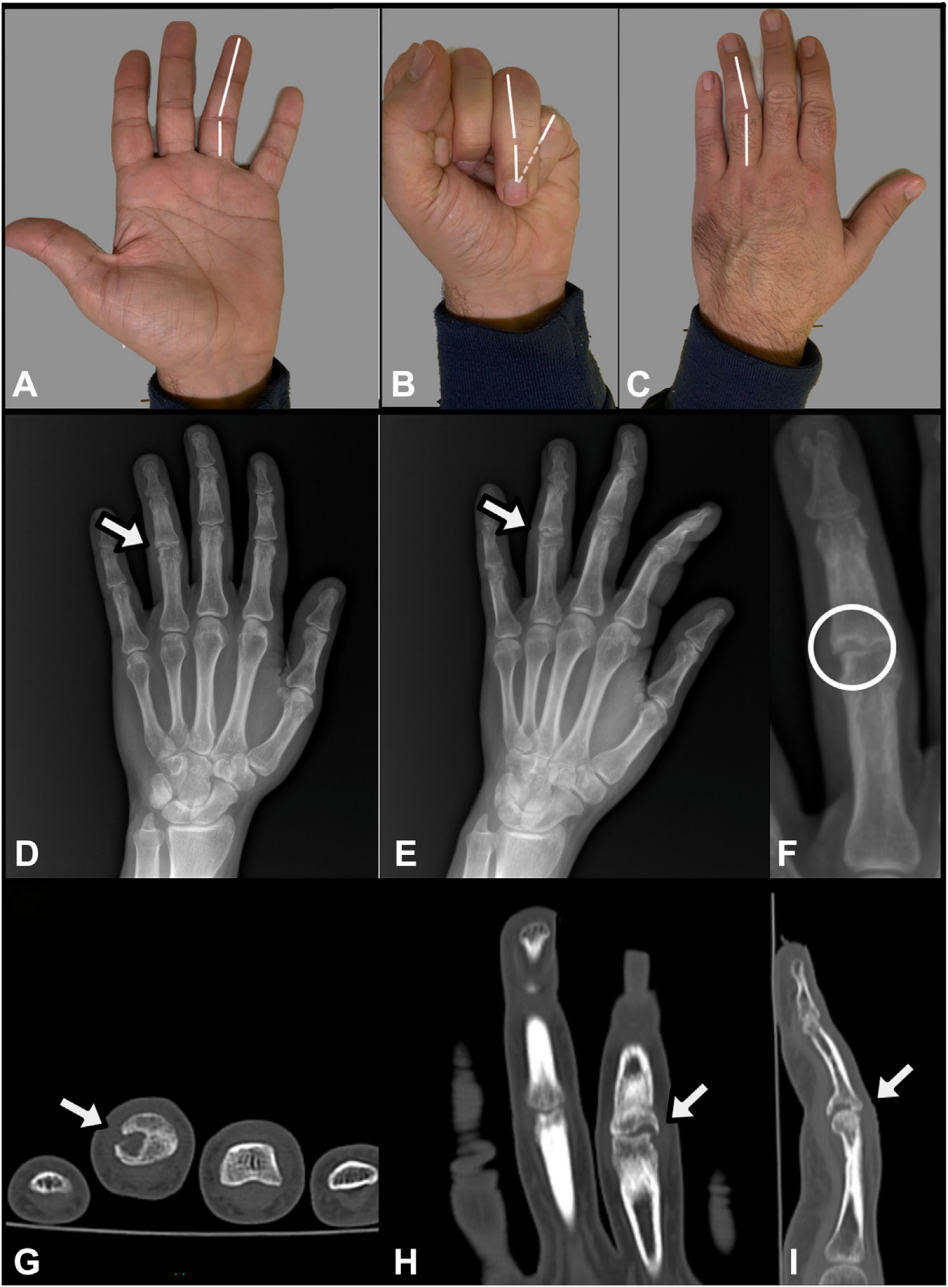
Preoperative assessment. A Palmar view of angular PIPJ ulnar deviation of 15° at full extended digits. B Palmar view of fourth finger overlapping fifth during flexion. C Dorsal view of fourth finger angular deviation. D Preoperative anteroposterior x-ray. We can notice PIPJ defect (arrow). E Preoperative oblique x-ray. F Detail of bone defect. G Preoperative CT scan axial view. H Preoperative CT scan transversal view. I Preoperative CT scan coronal view.
